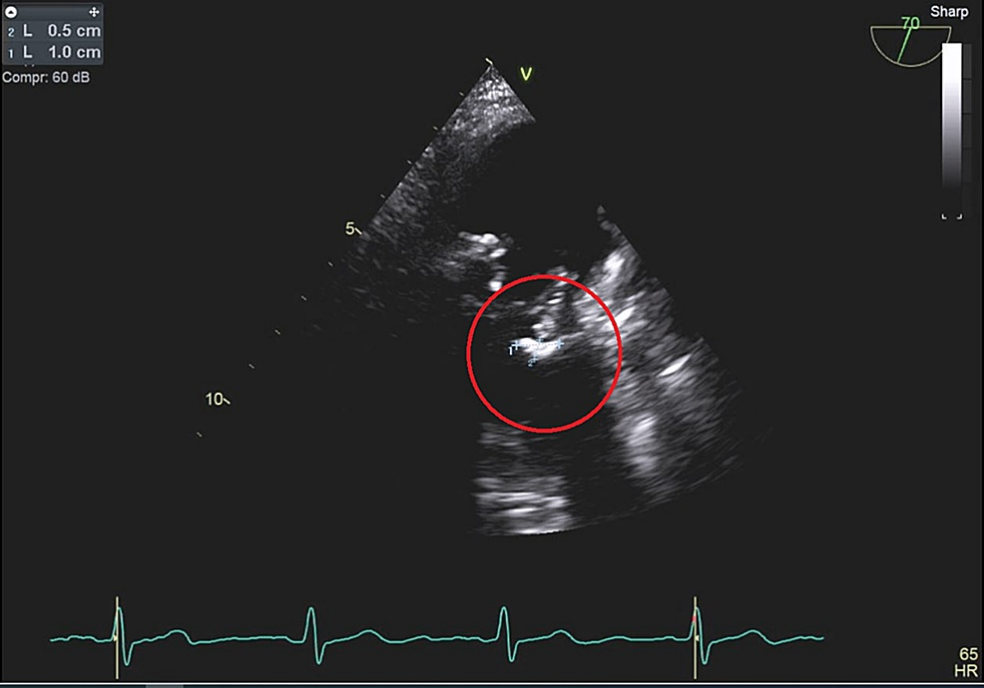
Transesophageal echocardiography (TEE) shows a bio-prosthetic mitral valve with a 1.0 x 0.5 cm vegetation on the posterior leaflet relative to the lateral wall of the left ventricle.
